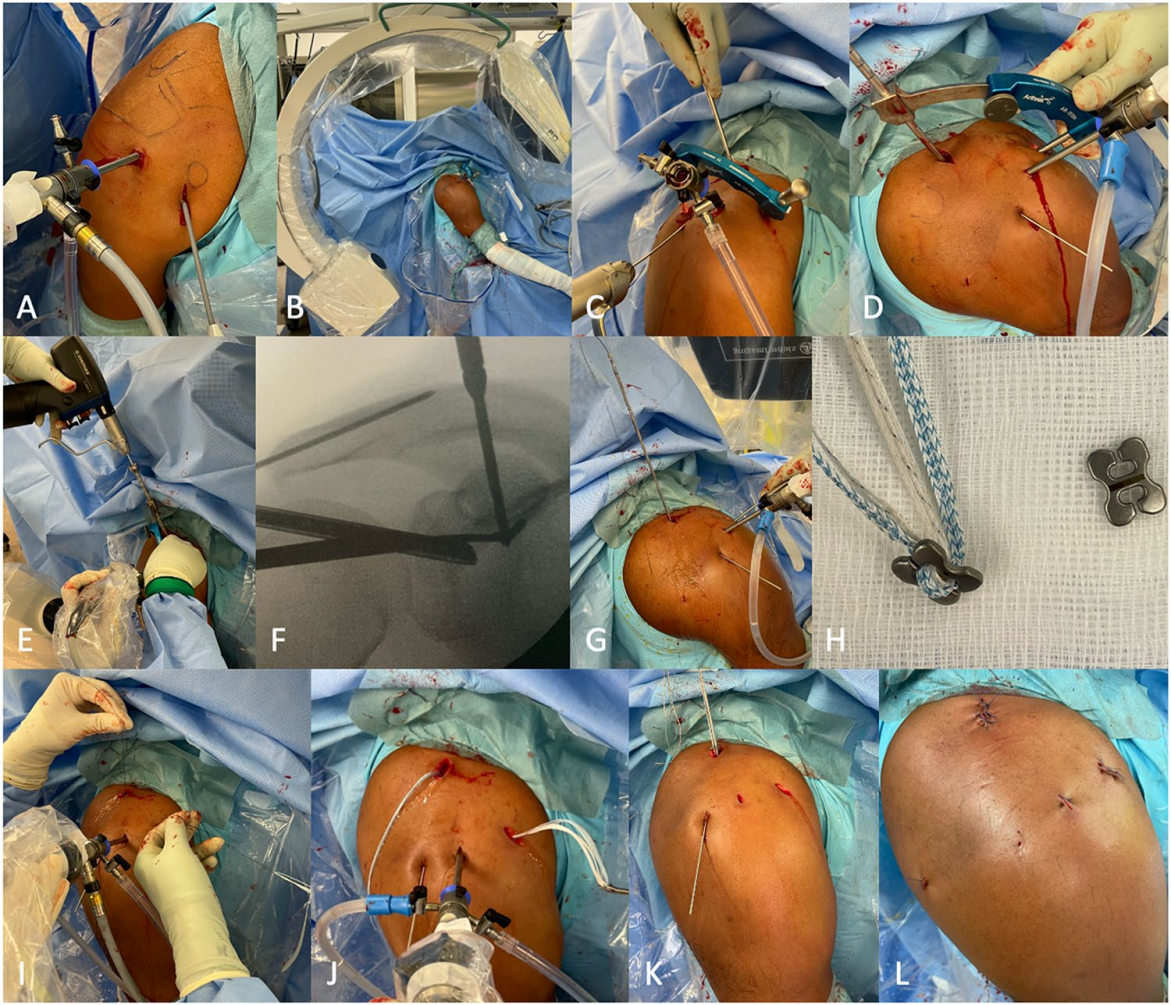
Arthroscopic-assisted technique. A After a standard diagnostic arthroscopy, the base of the coracoid is prepared via the anterior portal. B Under radiographic control, the ACJ is reduced and temporarily fixed with a K-wire (C). D Via the anterior porta, a guide instrument is placed underneath the coracoid base and via radiographic control on top of the clavicle. Using the guide instrument, transclavicular and transcoracoidal holes are drilled under radiographic and arthroscopic controls (F). G A guide wire is shuttled through the drill guide, the drill guide is then removed. H The pulley system (DogBone® attached to a TightRope®) is shuttled with the guide wire and placed at the coracoid base under arthroscopic control (I, J). K With another DogBone® Button, the vertical stabilization is secured. L Finally, an additional AC cerclage can be placed with the remaining K-wire. Afterward, the skin is closed with a monofilament suture
